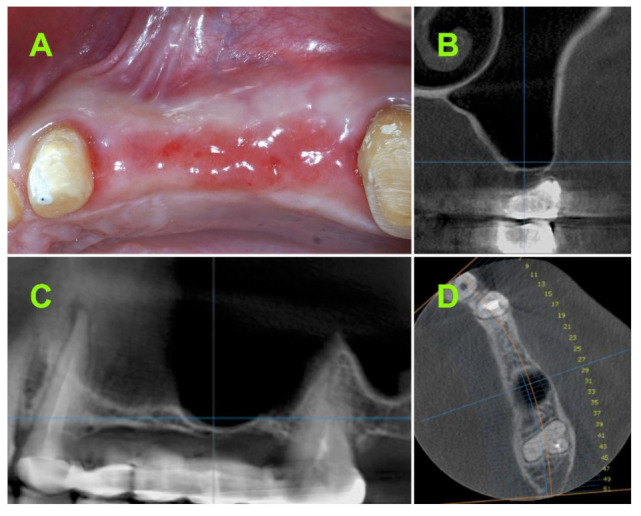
(A) Clinical aspect in the lateral area of upper maxilla; Pre-operative CBCT: (B) Coronal view; (C) sagittal view; (D) axial view.
